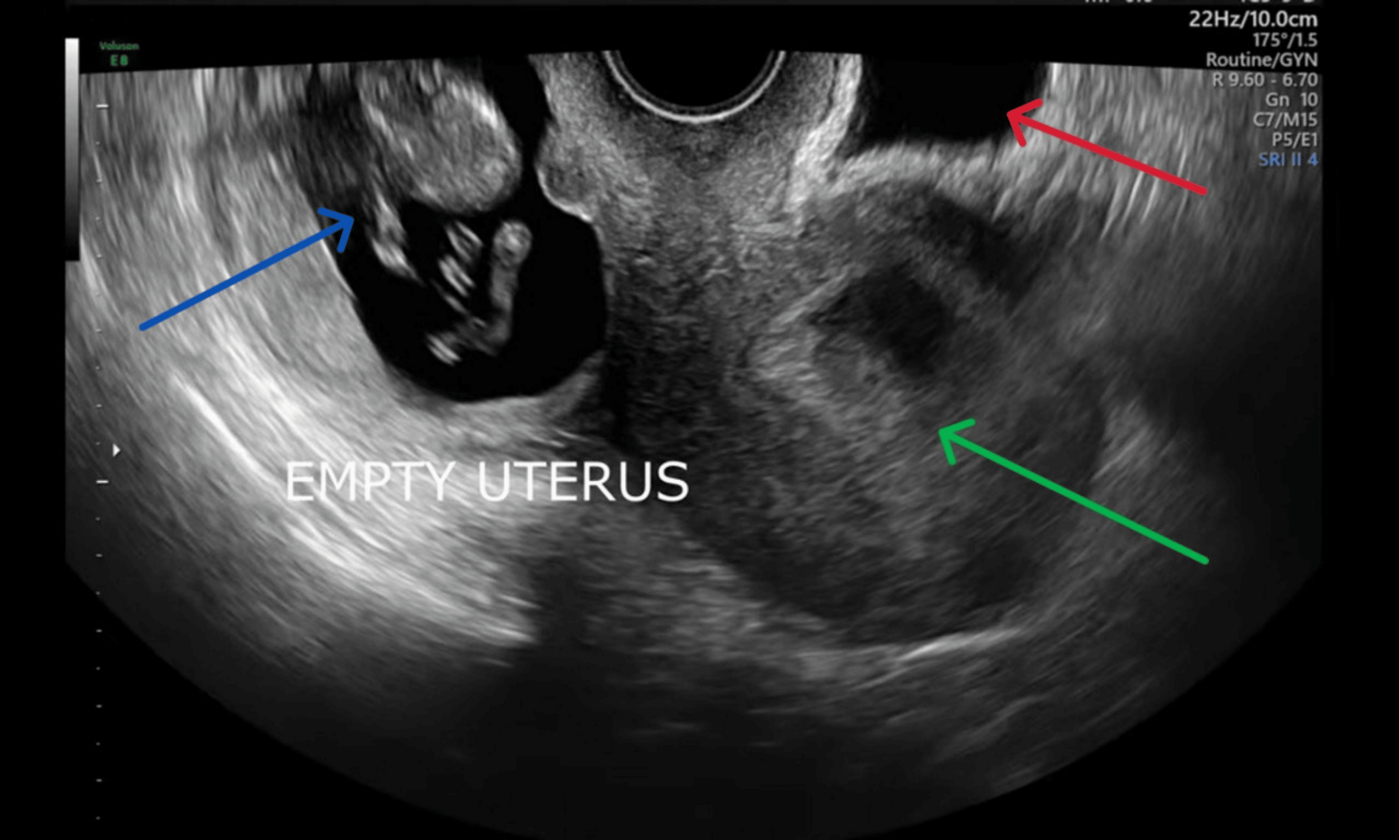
A transvaginal ultrasound shows empty uterus (green arrow) with right ovarian ectopic pregnancy (blue arrow) and the bladder (red arrow)
